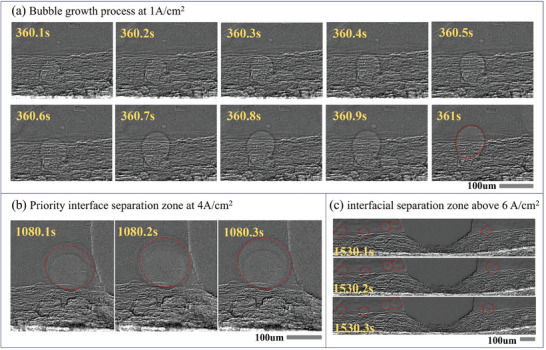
The cell cross‐sectional radiographs from continuous image stream for a) 1 A cm^−2^, b) 4 A cm^−2^, and c) 6 A cm^−2^ current density. (interfacial separation zones indicated by red lines). S1
